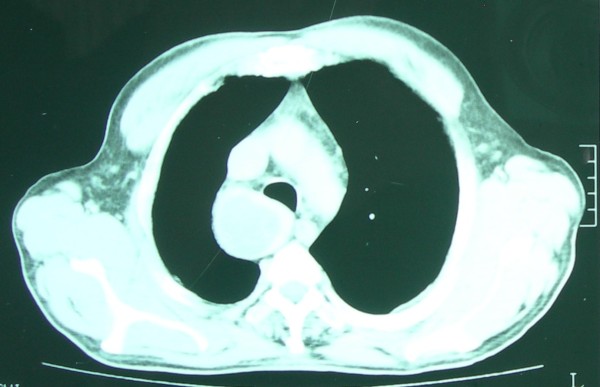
Computed tomographic scan reveals a well-encapsulated mass located at the right posterior mediastinum.
